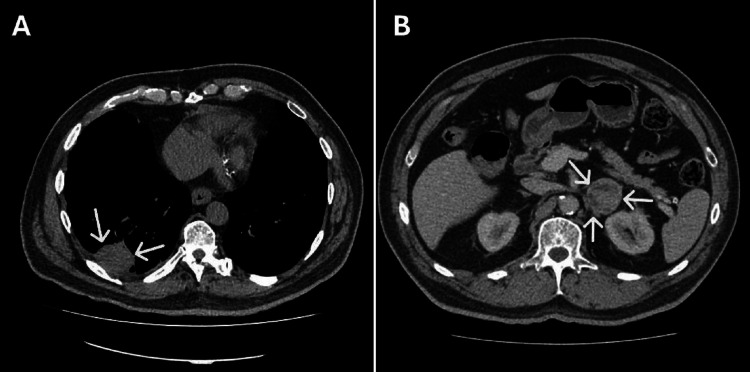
Radiological images of the chest and abdomen. A: High resolution computed tomography of the chest (transverse view) demonstrating a mass lesion measuring 7.0 cm x 6.1 cm (white arrows). B: Computed tomography scan of the adrenal glands (transverse view) demonstrating a large left inhomogeneous adrenal tumor measuring 4.3 cm x 4.9 cm (white arrows).
